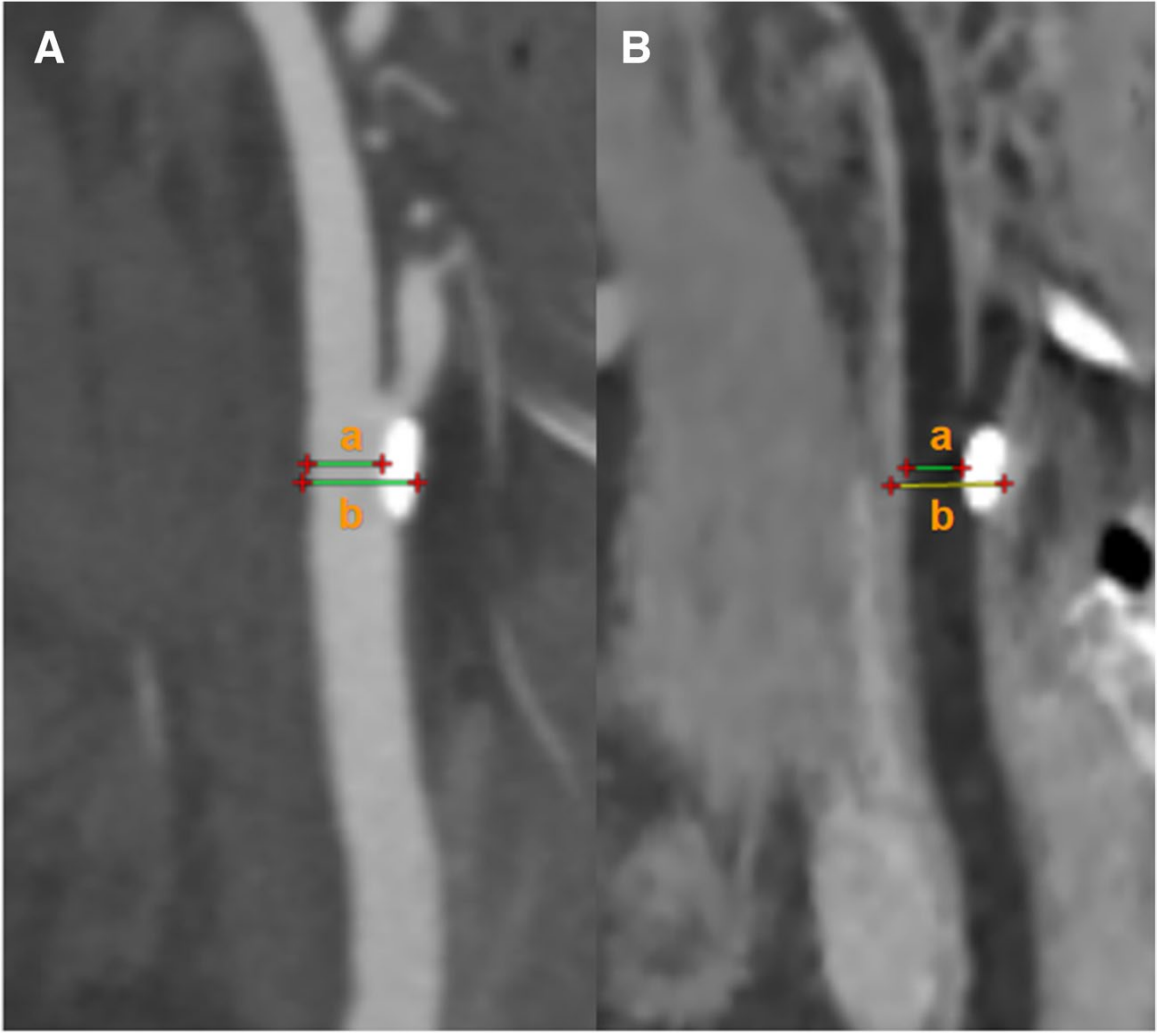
Measurement of stenosis rate. A CPR image of CTA. B CPR image of BBCT. There was a calcified plaque in the vessel wall of the common carotid artery. Stenosis rate = (1 − *a*/*b*)*100%
